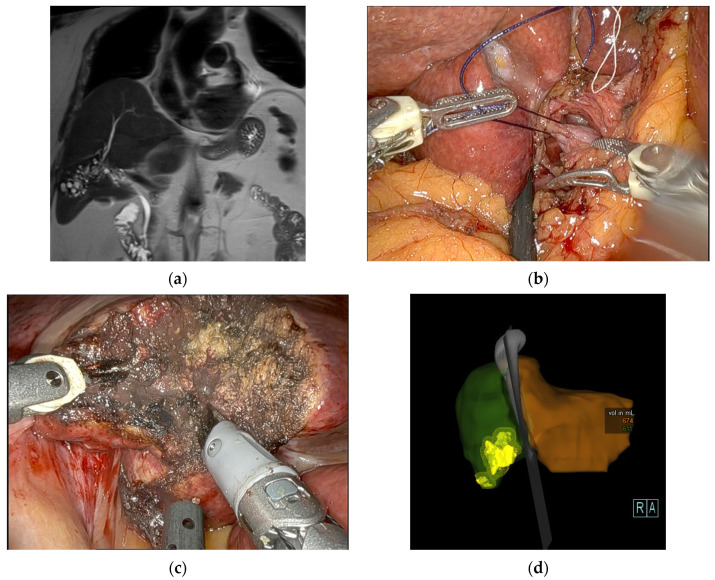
Patient with an AE lesion involving segments IVb/V/VIII with close contact to the right portal vein, who underwent an extended right hemihepatectomy by the ALPPS approach. (a) Preoperative MRI (T2-weighted coronal view); (b) Intraoperative image showing ligation of the right portal vein during the 1st step of ALPPS; (c) Intraoperative image showing the parenchymal dissection in S Ivb during the 1st step ALPPS; (d) CT liver volumetry 8 days after the 1st step ALPPS.
